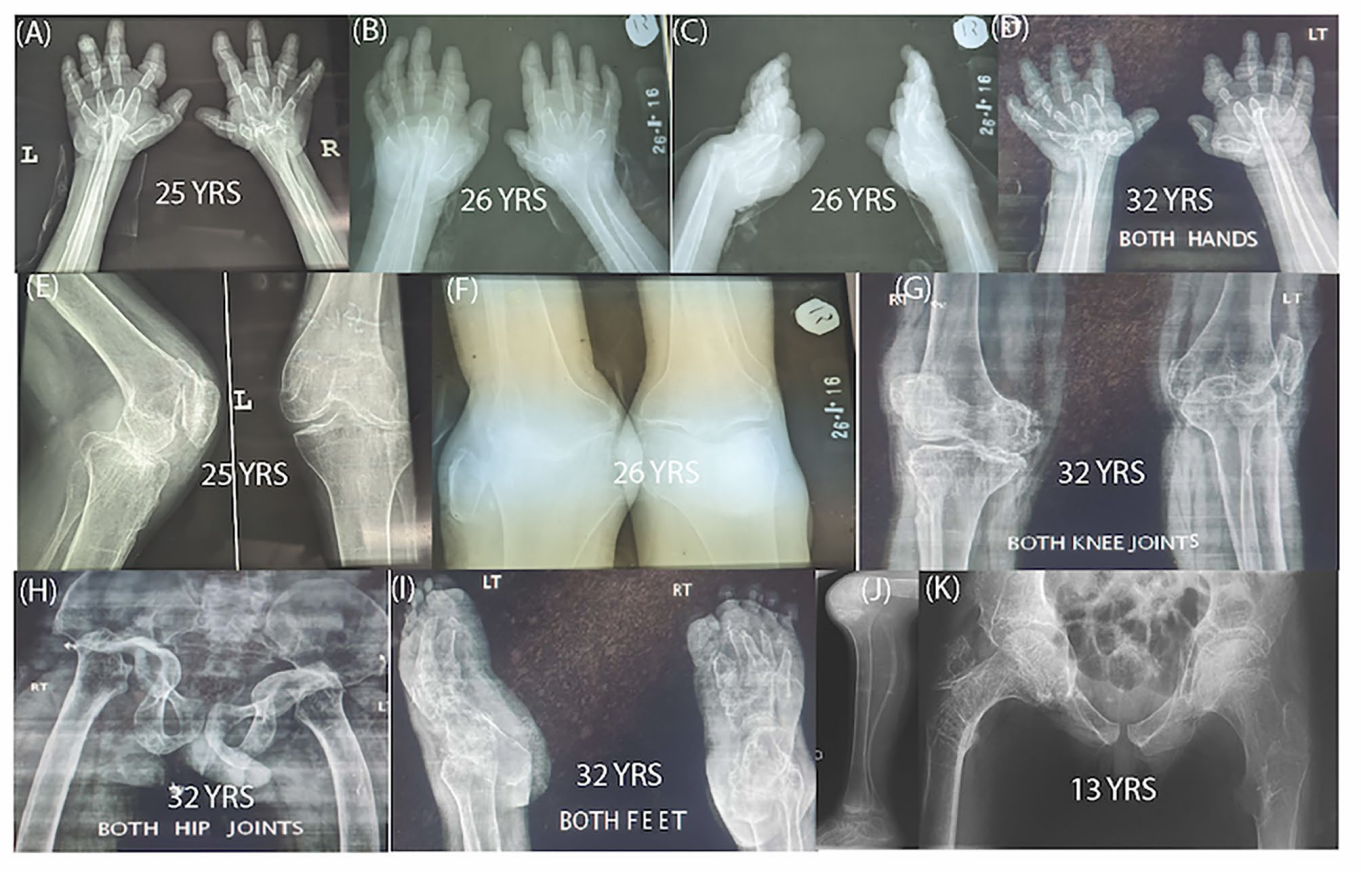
Radiographs of Family 1 and Family 2. Radiographs of patient IV:4 at age 25 years showing osteopenia, deformed wrist, distal radioulnar joint cortical thinning and bullet shaped metacarpals (B) Radiograph at age 26 shows increased osteolysis, poor visualization of carpal bones, cortical thinning of metacarpal bones and medullary expansion due to advanced osteopenia (C) Radiograph of the same patient at the age of 26 years, vertical view, showing protrusion of radius and ulna of the left hand (D) Radiograph of the same patient at age 32 showing complete osteolysis of carpal bones and abnormal shape of the metacarpal bones. Pencil-in cup deformity of most of proximal and distal interphalyngeal joints due to osteolysis is also visible (E) Radiograph of the knee joint at age 25 years showing marked narrowing of medial joint space of knee (F) At the age of 26 years, the knee showed more narrowing of space and progressive osteolysis (G) At the age of 32 years, the left knee joint shows destruction of the surfaces. The joint is locked; unable to move, with early osteoarthritic changes due to continuing osteolysis (H) Hip joint at the age of 32 years showing periarticular and generalized osteopenia osteonecrosis. There is complete destruction of femoral heads advanced protrusion acetabula bilaterally leading to deformed pelvic shape. Shepherd crock deformity of left femur is visible (I) Deformity and coalition of tarsal bones. (J, K) Radiographs of the Finnish male patient at age 13 years. Images show severe generalized demineralization and bowing of the very thin fibula. The knee and ankle joints show osteolytic changes and destruction of the joint surfaces. Proximal femurs are similarly osteopenic, femoral necks are thin and acetabuli and femoral epiphyses are irregular
